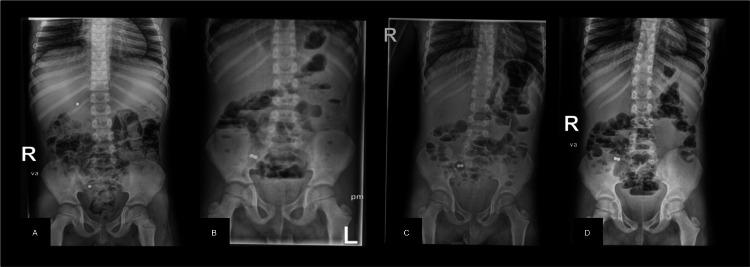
Abdominal radiographs at presentation and during observation. (A) Two magnetic foreign bodies (MFBs) seen separately on the initial radiograph. B) MFBs adhered to each other in the right lower quadrant. C–D) Serial radiographs obtained 8 and 24 hours later showed no progression of the MFBs.
